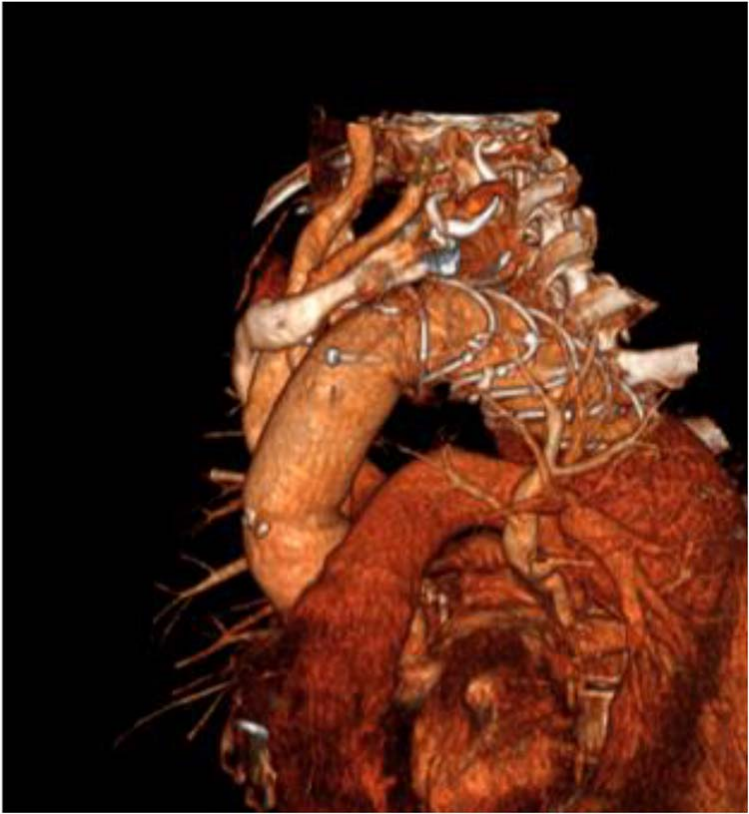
3D reconstructed CT aorta image showing successful FET implantation at zone 0.
